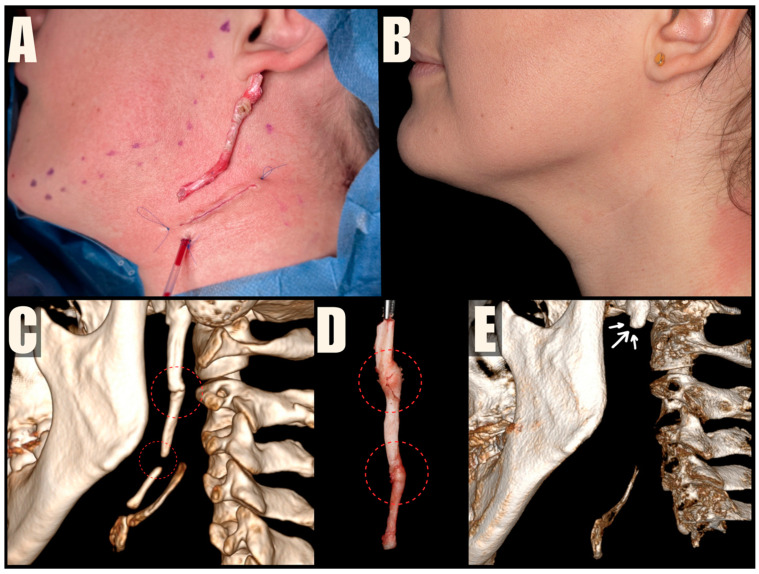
Case presentation of a patient with unilateral ES treated with MICS. (A) Intraoperative image of the removed elongated SP, with sutured wound and Redon drainage. (B) Scar after 6-month follow-up. (C) Preoperative CT scan with elongated SP. (D) Removed SP, classified as Langlais type 2; areas of calcification of the stylohyoid ligament marked with a dashed line. (E) Postoperative CBCT scan showing residual SP, indicated by arrows.
